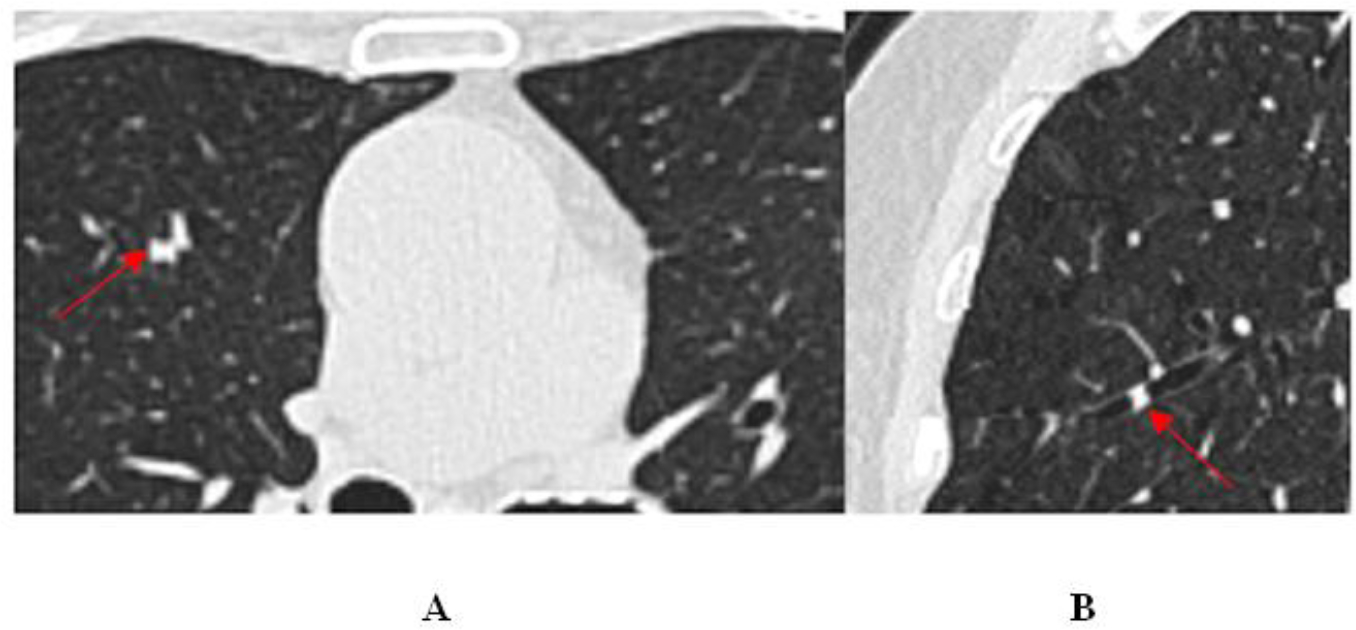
A) Axial CT image showing a mucus plug occluding an airway (arrow) in the right upper lobe; B) The same mucus plug in the sagittal plane.
